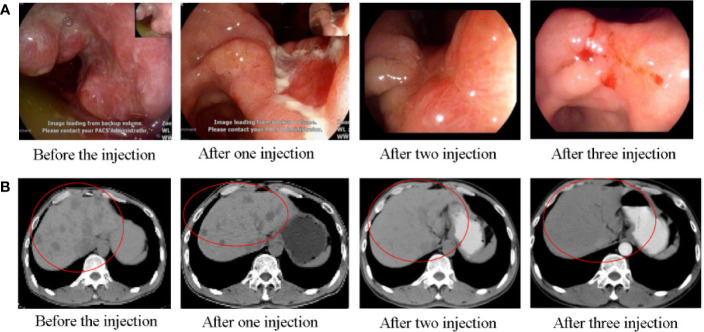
A 51-year-old male patient was diagnosed with T2N1M1 stage gastric carcinoma (GC), involving the entire gastric angle and anterior wall of lesser curvature of gastric antrum. (A) The gastroscopy showed that there were huge ulcer-like tumors in the whole gastric angle and the anterior wall of the lesser curvature of the gastric antrum. The bottom of the tumor was uneven, nodular, and covered with white moss, and the edge was raised, with erosion, hyperemia, edema, poor peristalsis, and hard texture. After treatment, the huge ulcer-like tumor was reduced and healed, and the mucosa was gathered, the edge was raised, congestion and edema were reduced, and the tumor volume was significantly reduced. (B) Contrast-enhanced CT of the upper abdomen, before endoscopic injection treatment, revealed multiple low-density foci in the liver parenchyma before endoscopic injection, with unclear boundaries, the largest of which was about 2.5 cm in diameter. After the treatment, CT examination showed that the multiple metastases in the liver were significantly reduced and smaller than before and some of the low-density lesions in the liver disappeared.
